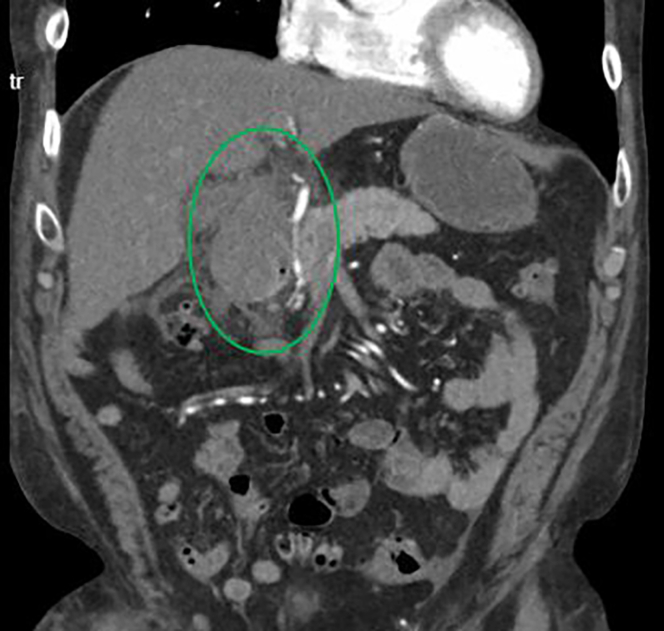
Computed tomography angiography coronal view of the intramural duodenal hematoma *(green circle)*.
